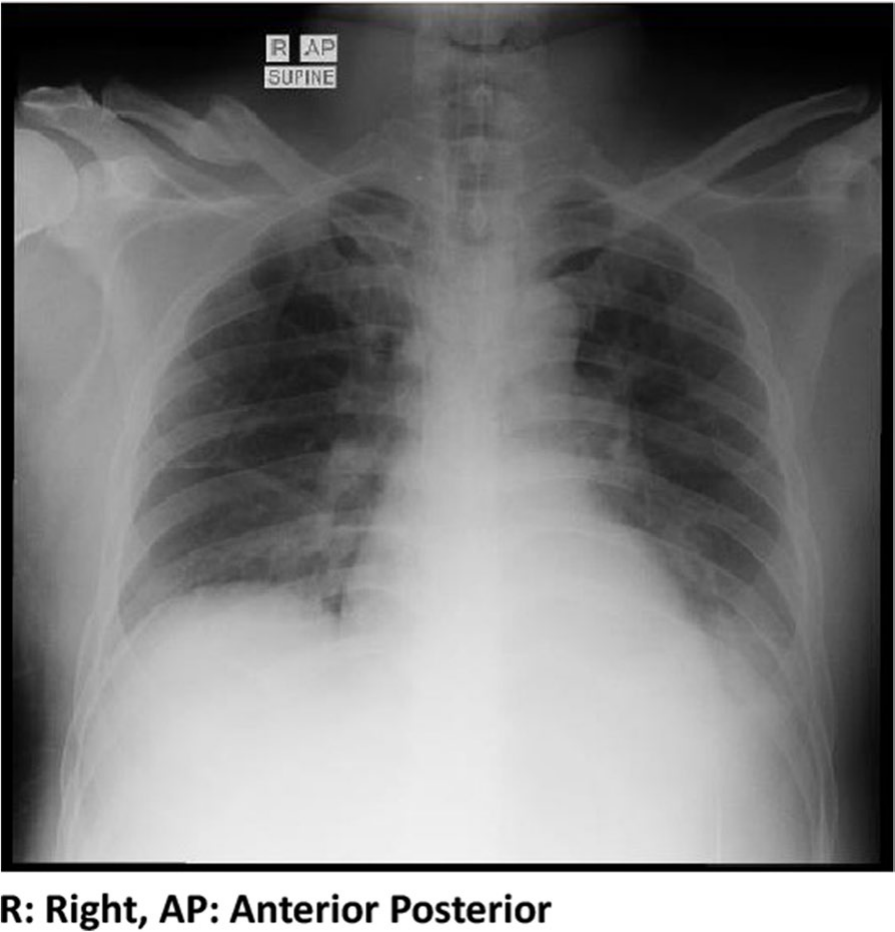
Chest X-ray showing bilateral pulmonary infiltrate with left pleural effusion
